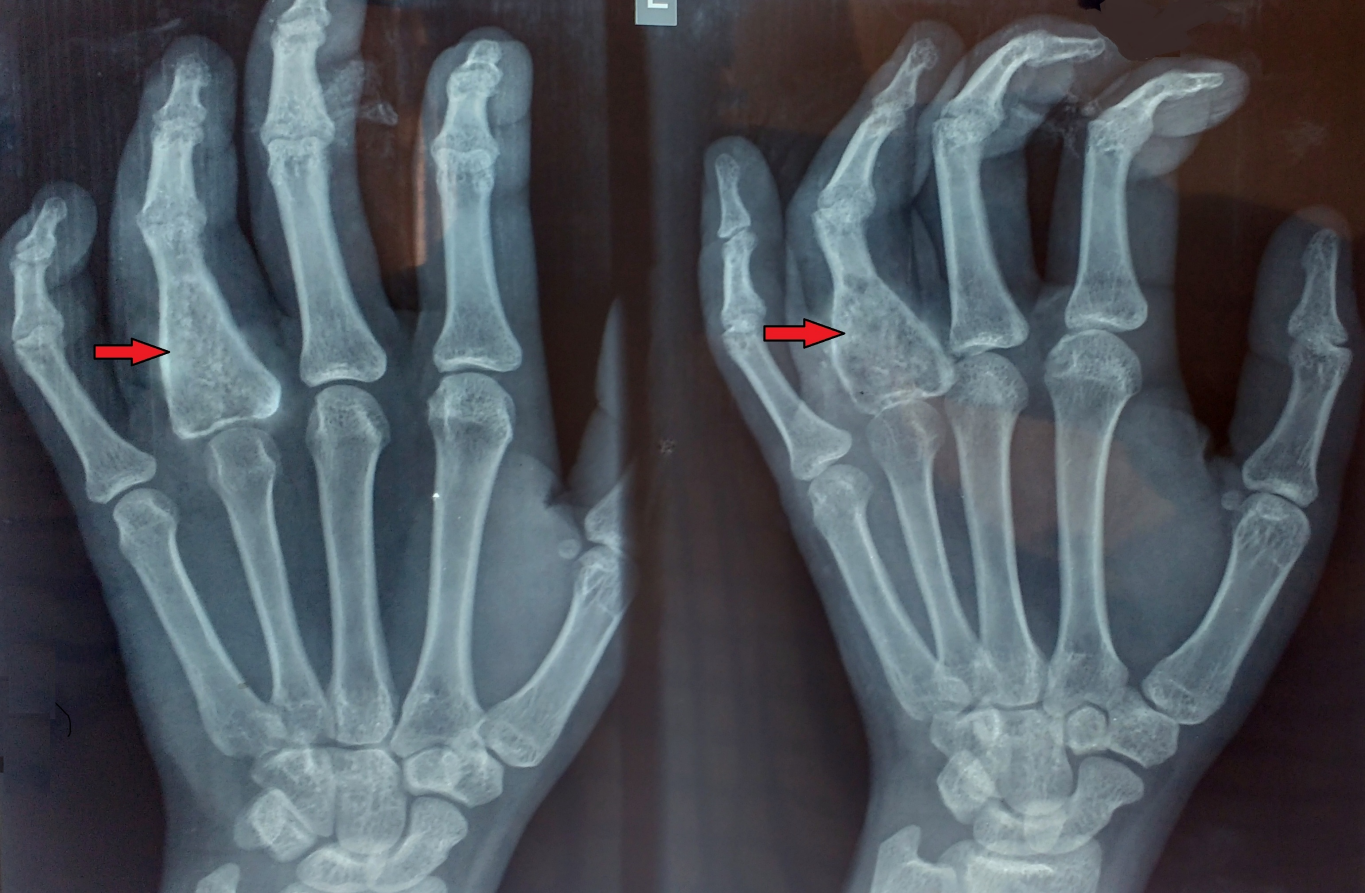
Follow-up radiograph at two years Shows good incorporation of the bone graft and no signs of recurrence
